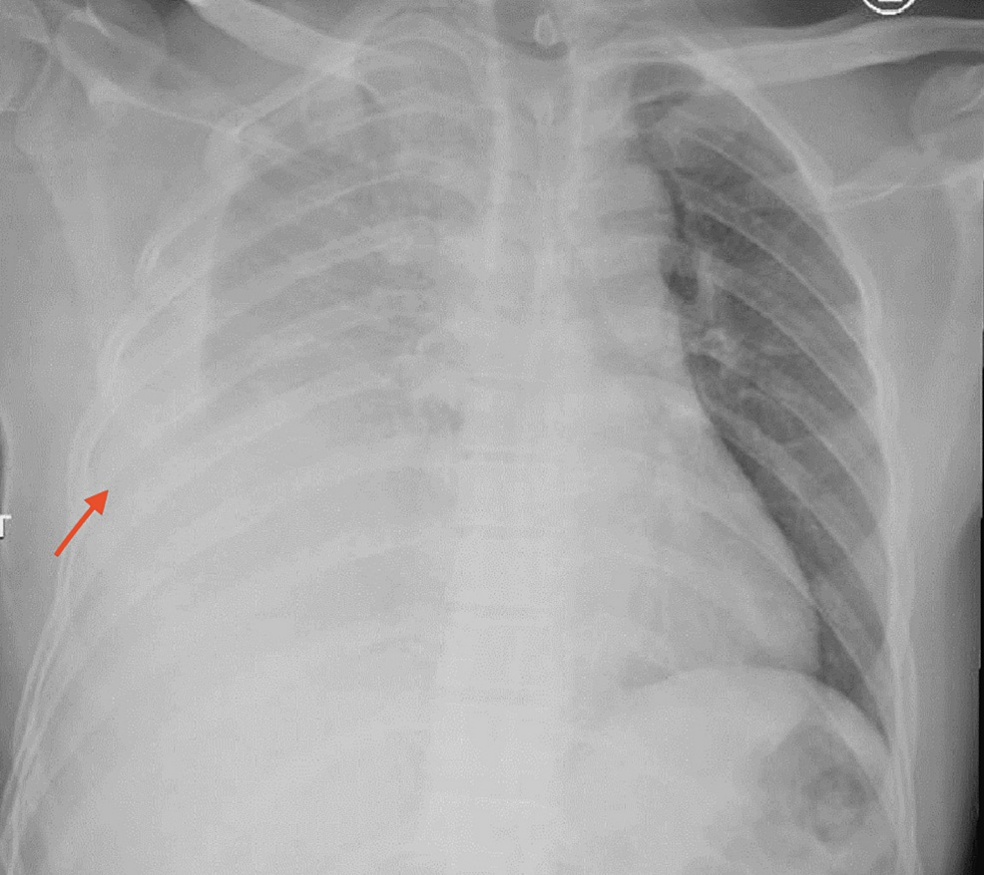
Initial chest X-ray showing right-sided hemothorax and elevated right diaphragm.
